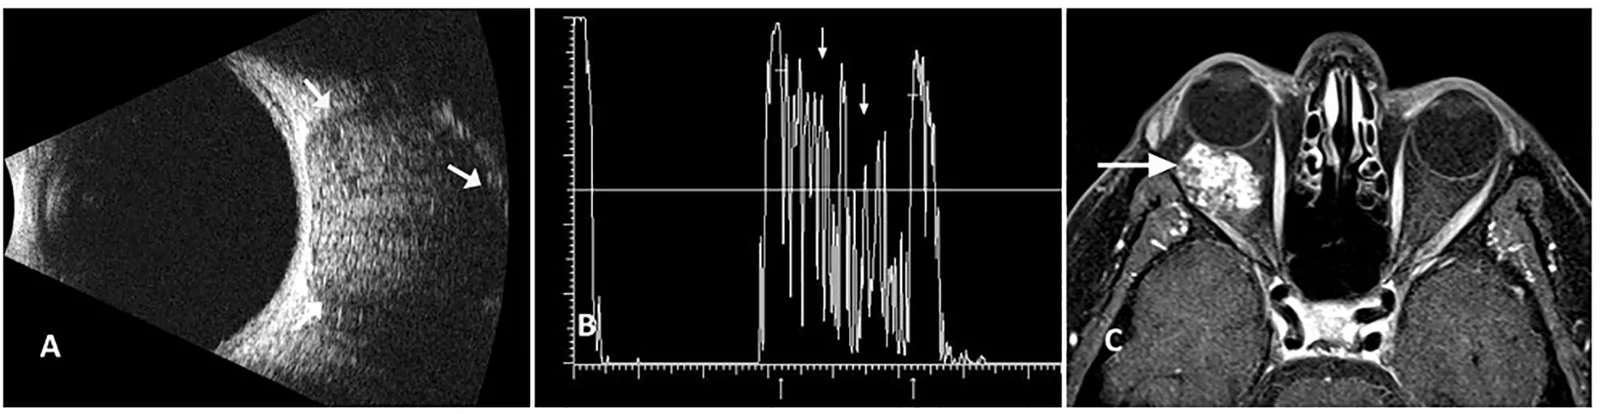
Cavernous Venous Malformation. (A) Transverse B-scan shows a large, well-outlined, oval shape, homogeneous orbital lesion (arrows). (B) A-scan shows high reflectivity and moderate sound attenuation (arrows). (C) Axial post-contrast T1-weighted MRI shows an intraconal mass (arrow).
